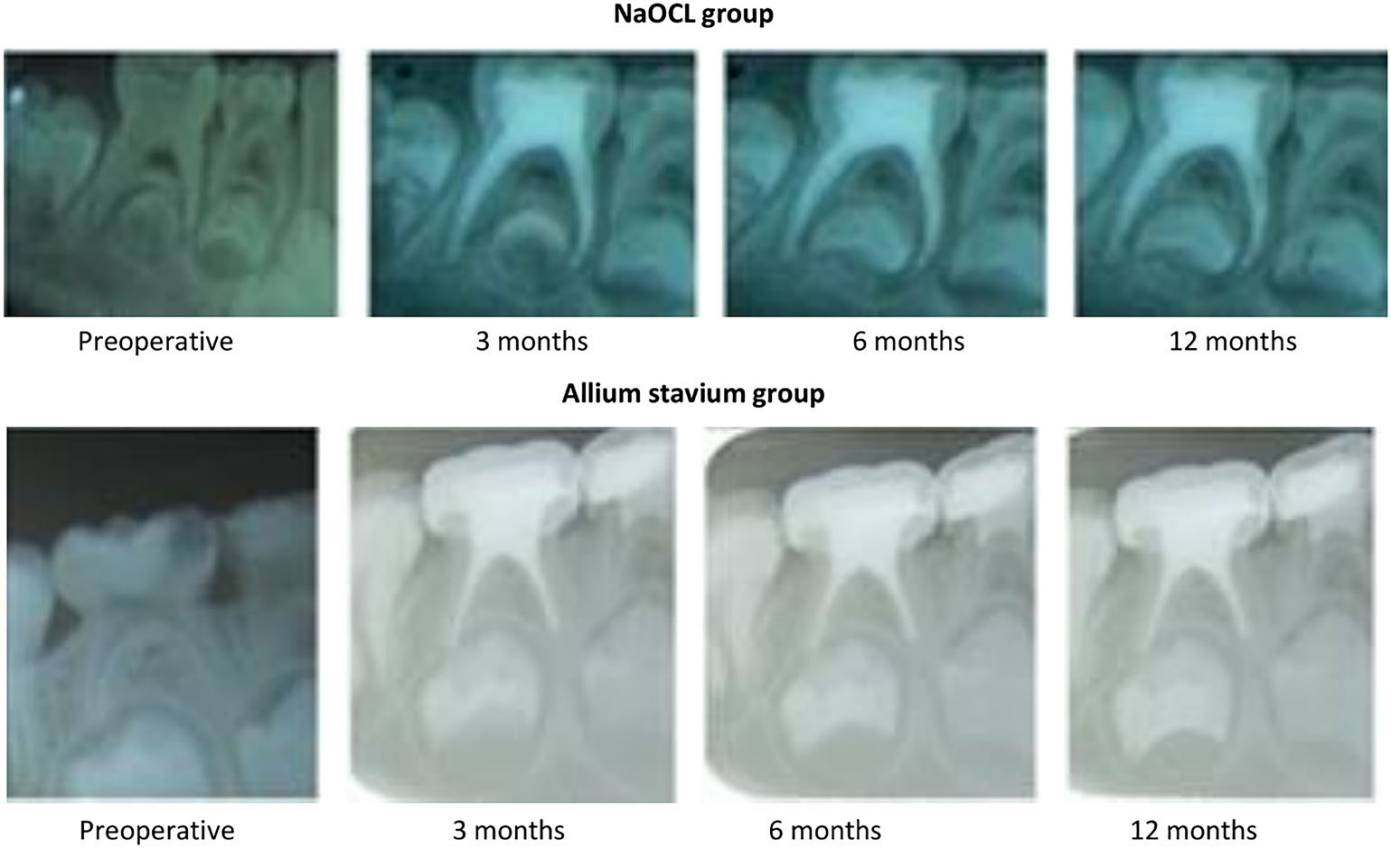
Periapical radiographs show successful pulpectomy of Allium sativum extract and sodium hypochlorite in the lower right second primary molars
